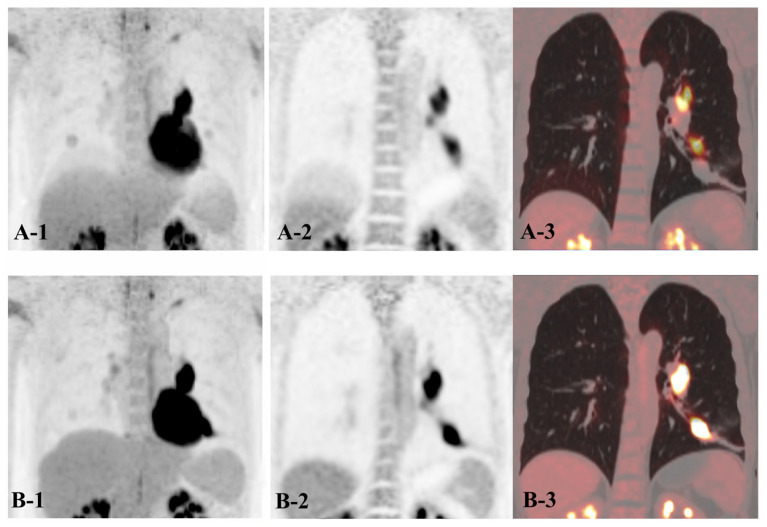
The scan CB image of a 47-year-old patient diagnosed with small cell lung cancer in the left lower lobe accompanied by left hilar lymph node metastasis. A denotes NMC reconstruction (A-1: PET Maximum intensity projection, A-2: PET coronal view, and A-3: PET/CT fusion image); the visual assessment yields poor or moderate scores on respiratory motion of the liver (0 point), PET/CT alignment (1 point), and lesion clarity (1 point). B denotes RMC reconstruction (B-1: PET Maximum intensity projection, B-2: PET coronal view, and B-3: PET/CT fusion image); the visual assessment yields good scores on respiratory motion of the liver (2 points), PET/CT alignment (2 points), and lesion clarity (2 points).
